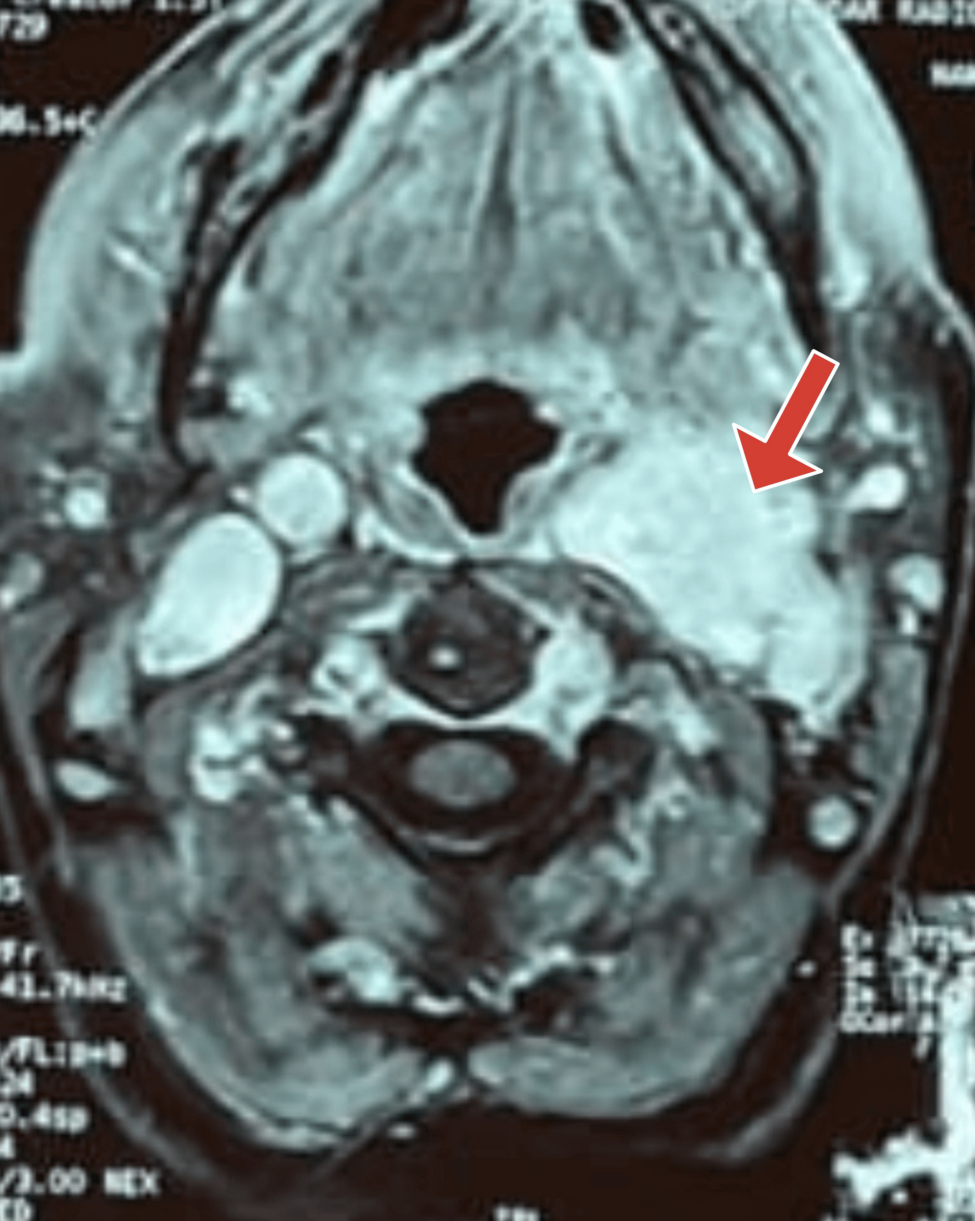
CT scan of the neck showing a soft tissue mass (red arrow) at the left carotid bifurcation splaying the ICA and ECA ICA: Internal carotid artery; ECA: External carotid artery
